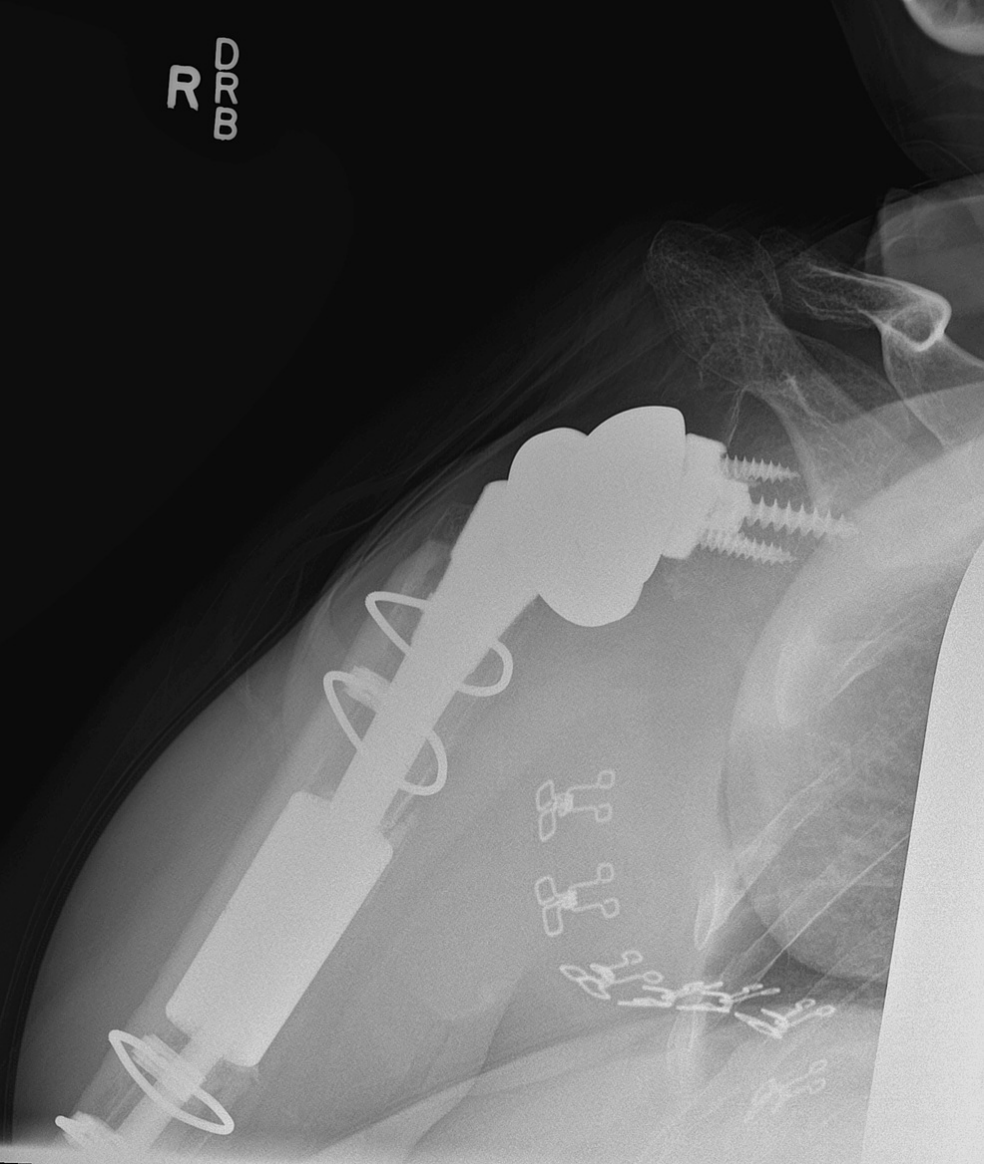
AP oblique radiograph of the right shoulder at 12-month follow-up. AP: anteroposterior
